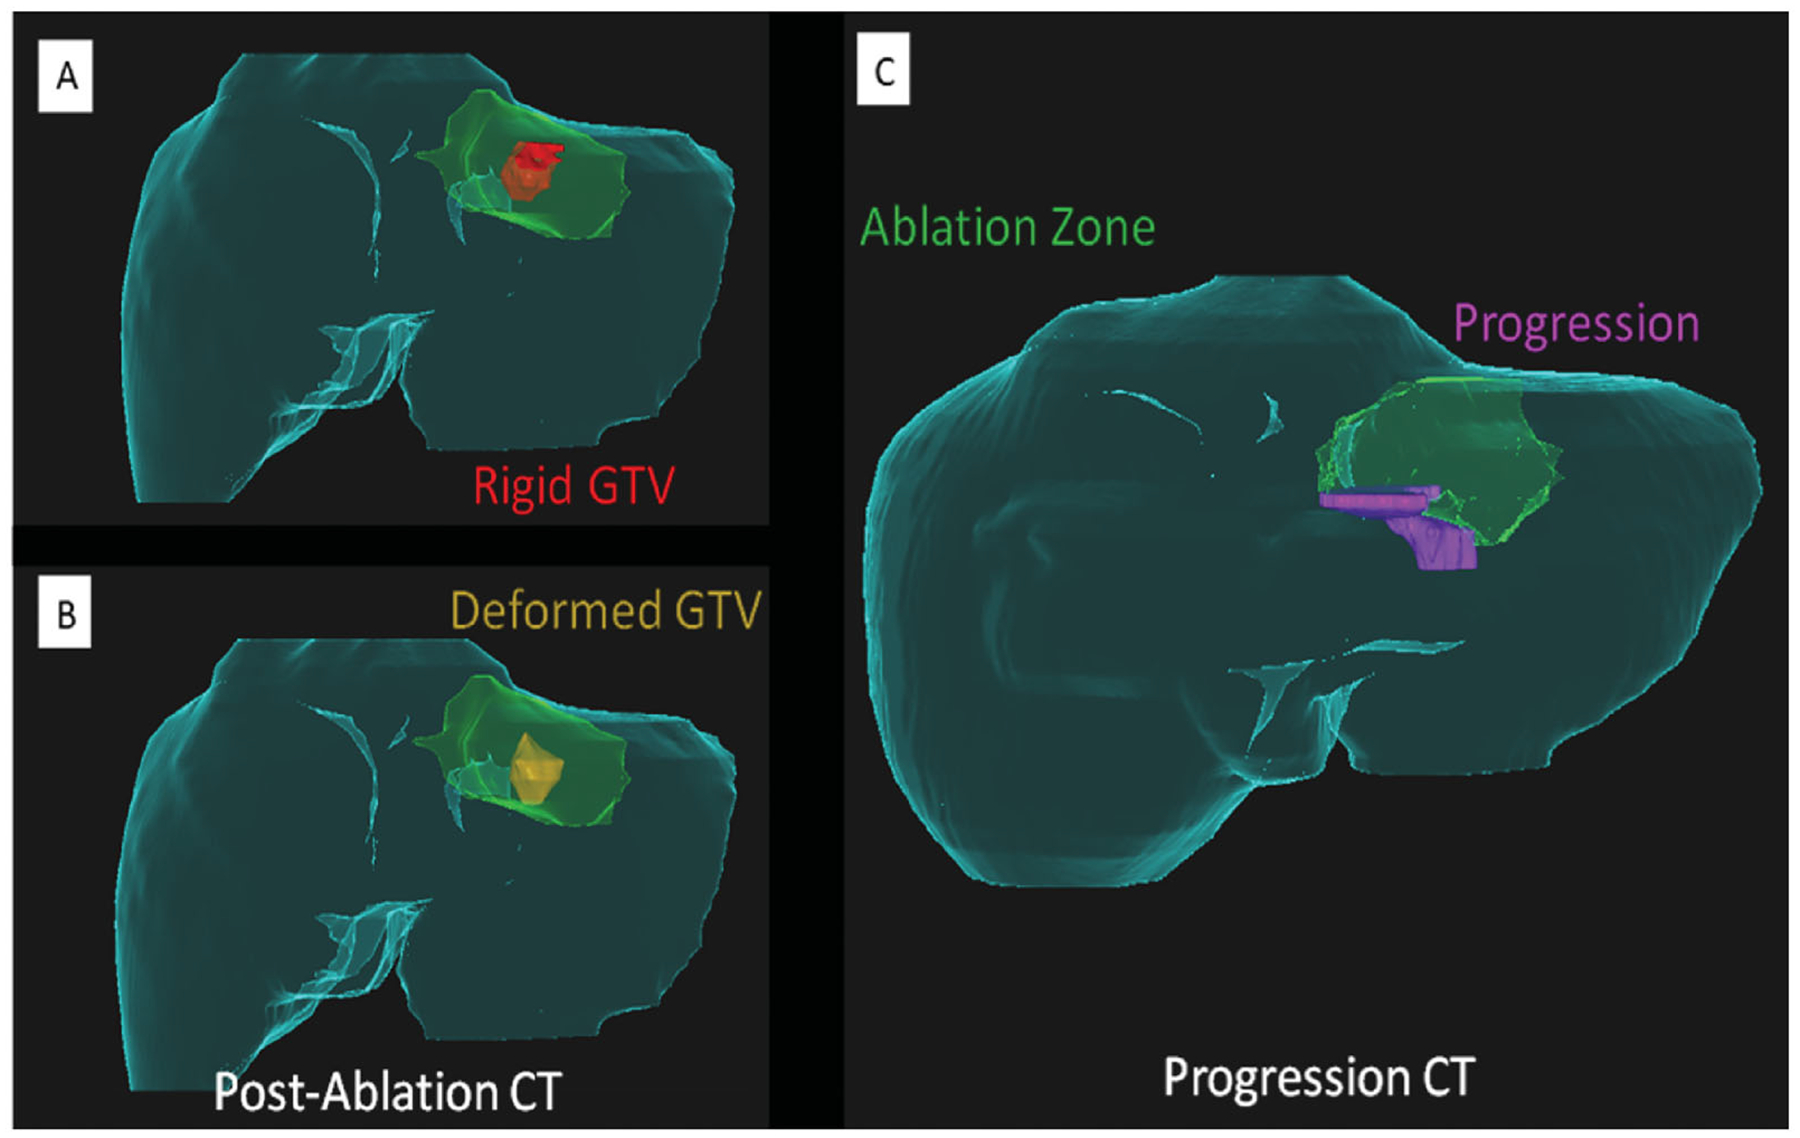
Importance of a deformable registration model use for assessing ablation margins. (A) 3 D CT reconstructionu using a rigid registration model. Gross tumor volume (GTV, red) is depicted onto the postablation CT maps within the ablation zone (green area); (B) 3 D CT reconstruction using a deformable registration model depicting GTV (yellow) in close contact with the ablation zone margin (green area) caudally, reflecting insuficient ablation margins (2 mm) at that region; (C) 6-month postablation 3 D CT reconstruction using a deformable registration depicting local tumor progression (purple area) and the caudal aspect of the ablation zone (green area), which was deemed to have insufficient ablation margins per biomechanical deformable registration model. Courtesy: Brian Anderson, PhD and Kristy Brock, PhD.
